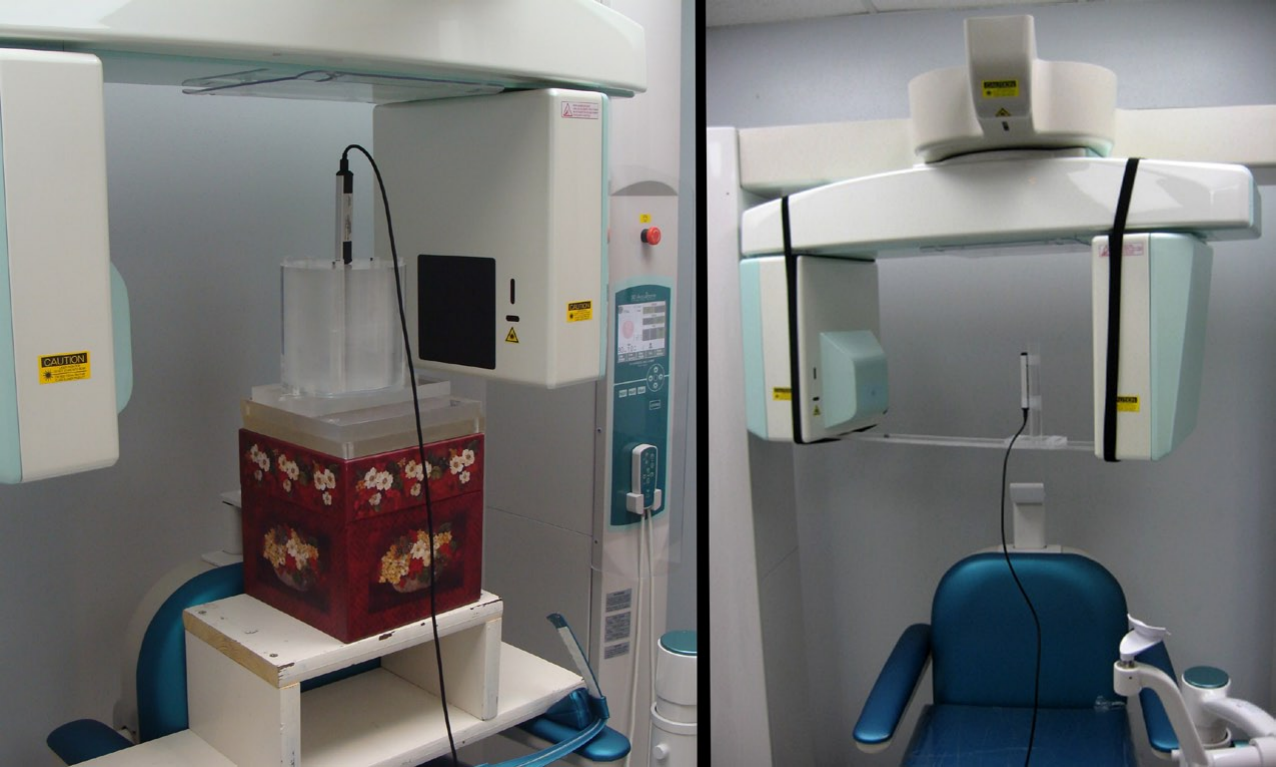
Setup for measuring HVL at isocenter for the Morita 3D Accuitomo cone‐beam CT unit using an Unfors dosimeter (right), and CTDI on same cone‐beam unit (left).
